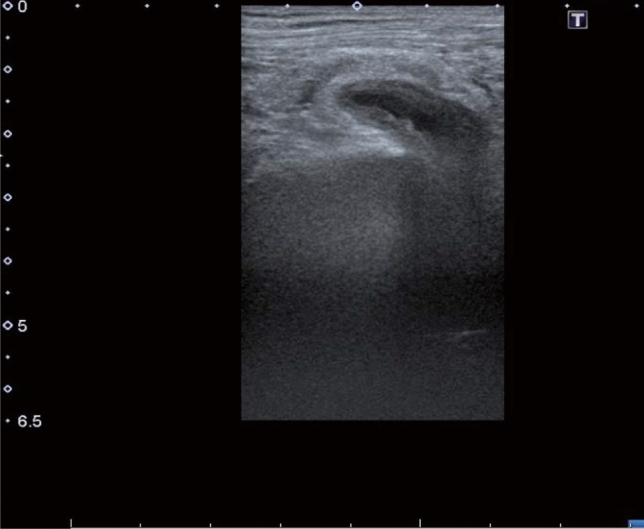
Ultrasound showed the incarcerated small intestine.
